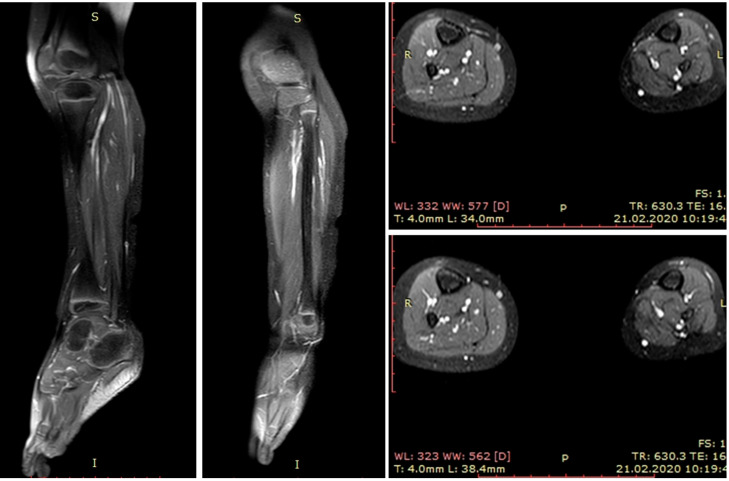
Magnetic resonance imaging (MRI) at the two years follow up. Reconstructed superficial compartment with functional vastus muscle flap can be visualized and no RMS (rhabdomyosarcoma) recurrence present. The healthy right size approximately 30% bigger comparing reconstructed triceps muscles.
